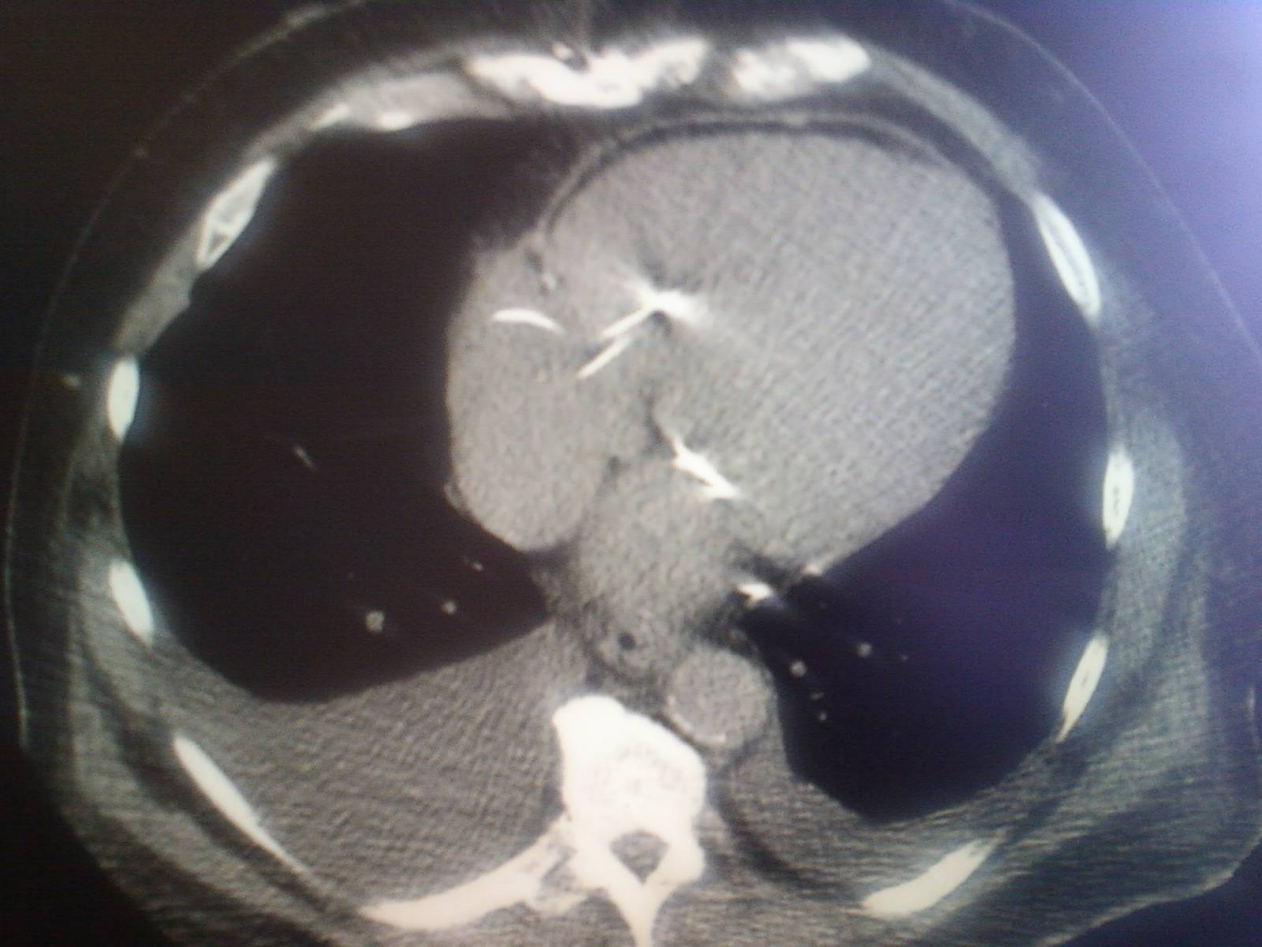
Preoperative Chest CT Scan.
